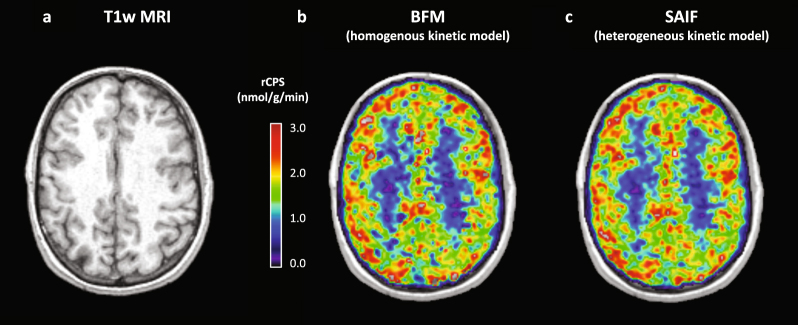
rCPS estimated with BFM and SAIF. (a) MRI T1-weighted image. (b) rCPS parametric map obtained with BFM (under the hypothesis of a homogenous tissue kinetic model). (c) rCPS estimated with SAIF (under the hypothesis of a heterogeneous tissue kinetic model). Data are from a single 21 year old male subject who underwent a L-[1-^11^C]leucine PET study with the High Resolution Research Tomograph (injected dose 0.39 mCi/kg).
